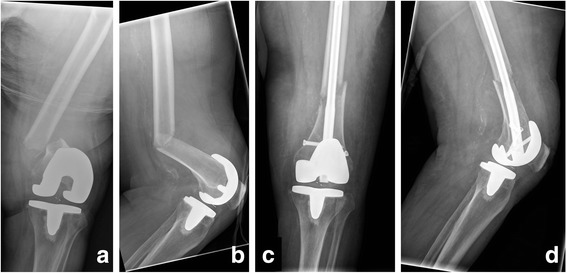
X-ray images of a 92-year-old woman with a periprosthetic fracture of the left femur after cruciate retaining (CR) bicondylar TKA (type A I); a: anterior-posterior and b: lateral radiation path with representation of the fracture before supply; c: anterior-posterior and d: lateral radiation path after reconstruction and supply by retrograde nail osteosynthesis
